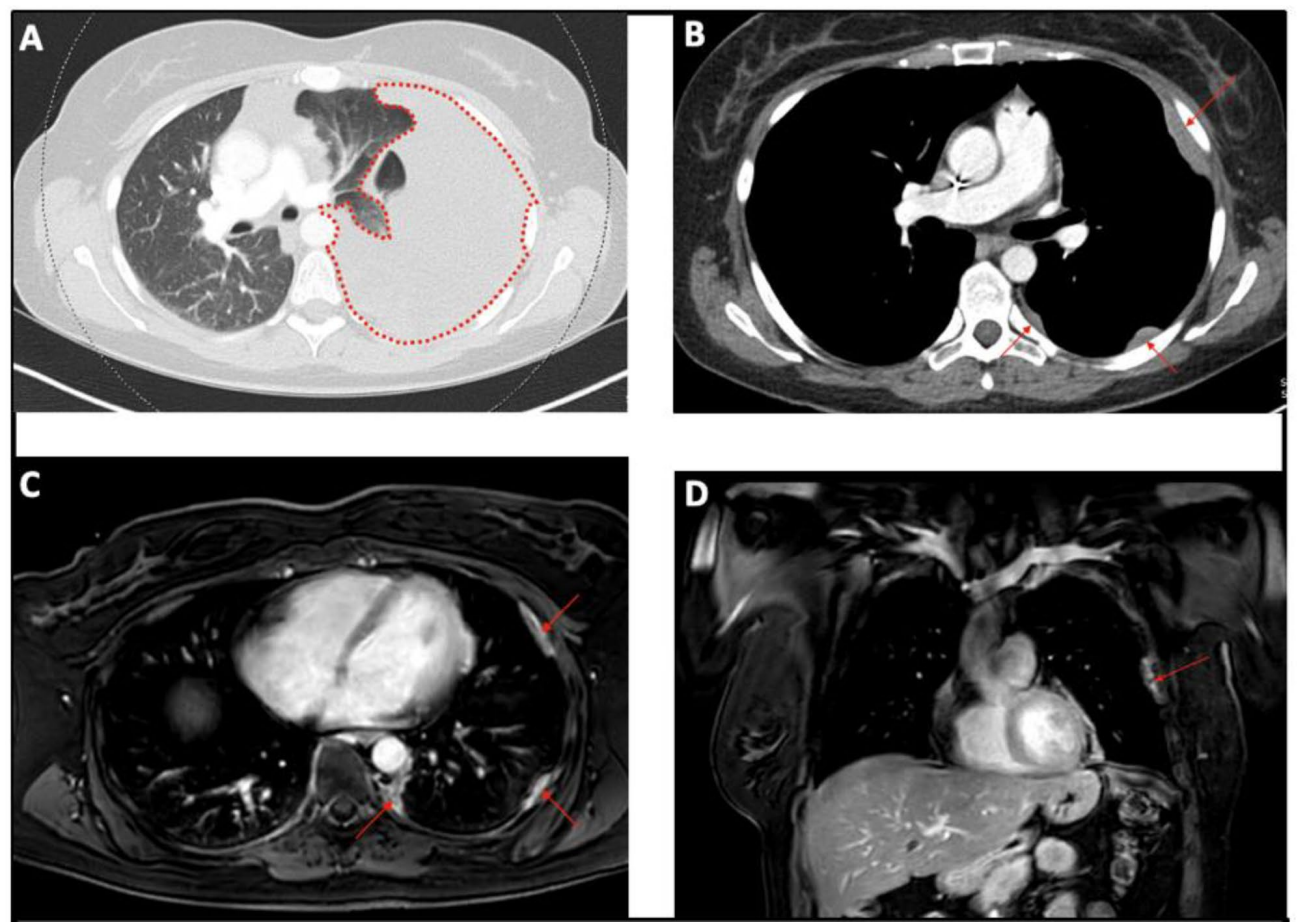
(A) Chest CT showing a large left sided pleural effusion; (B) Chest CT after thoracocentesis revealing multiple pleural nodules; (C) Preoperative chest MRI showing residual pleural disease post-chemotherapy; (D) Preoperative chest MRI showing residual pleural disease post chemotherapy
